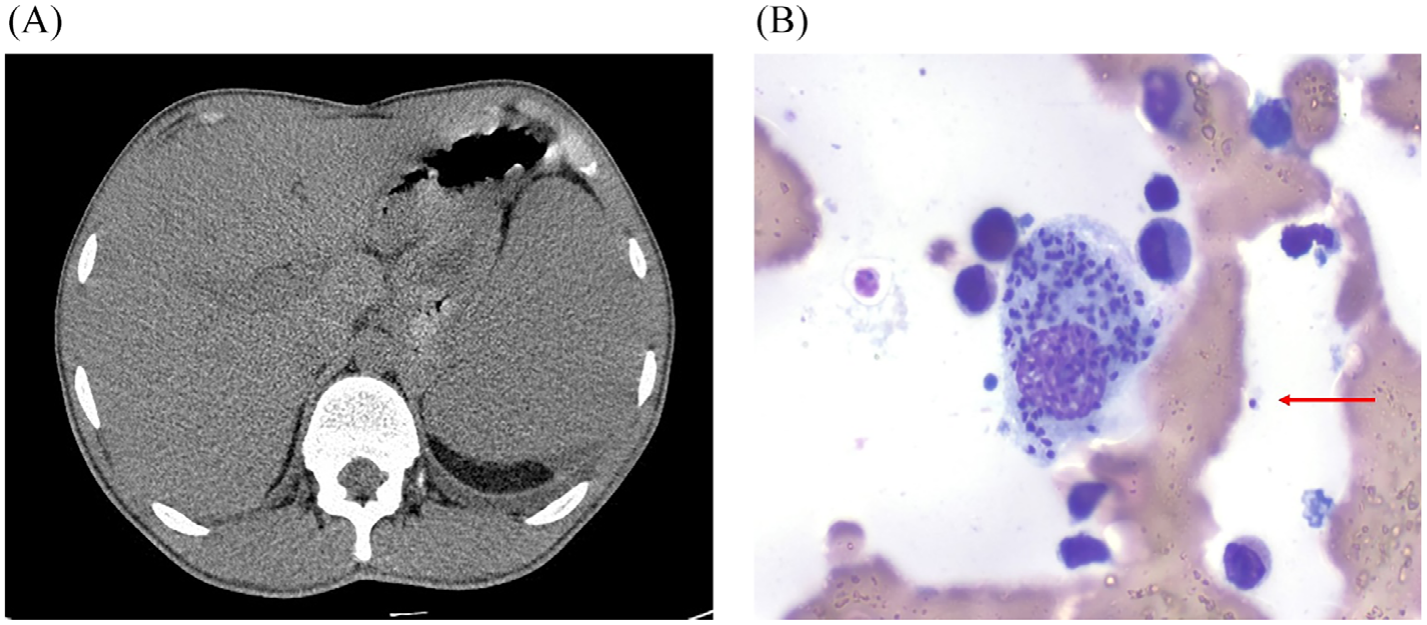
(A): CT scan showing an enlarged spleen. (B): Bone marrow smear showing several amastigotes engulfed by a macrophage. A free amastigote can also be seen (red arrow)
